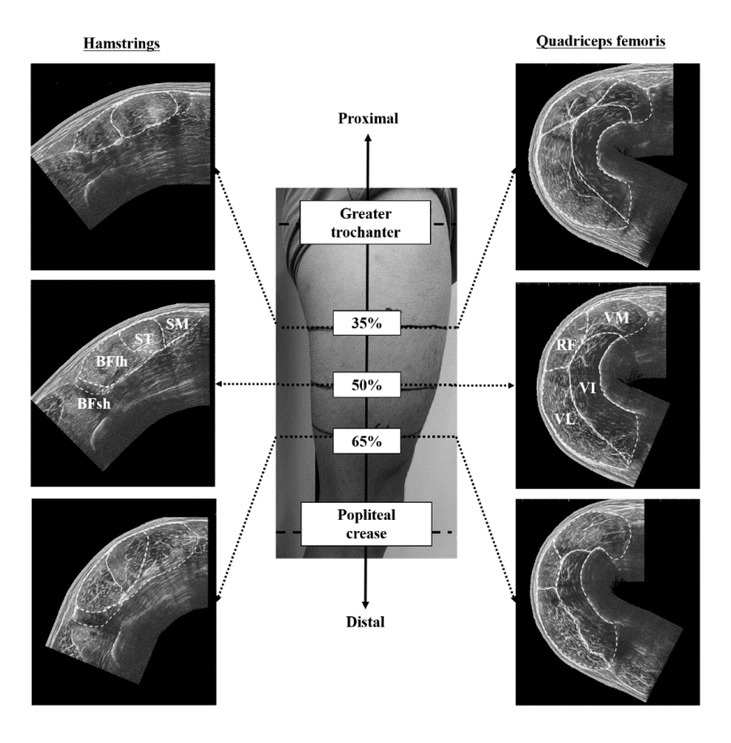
Measurement locations of the anatomical cross-sectional area and example of the ultrasound images in the thigh muscles. The white broken line indicates the outline of individual muscles of the hamstring and quadriceps femoris. BFlh, biceps femoris long head; BFsh, biceps femoris short head; ST, semitendinosus; SM, semimembranosus; RF, rectus femoris; VL, vastus lateralis; VM, vastus medialis; VI, vastus intermedius.
